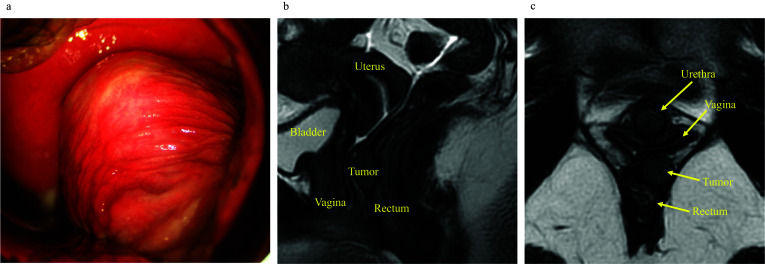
Preoperative findings. a The tumor observed transvaginally. b Preoperative magnetic resonance imaging findings (T2, sagittal). c Preoperative magnetic resonance imaging findings (T2, axial)
